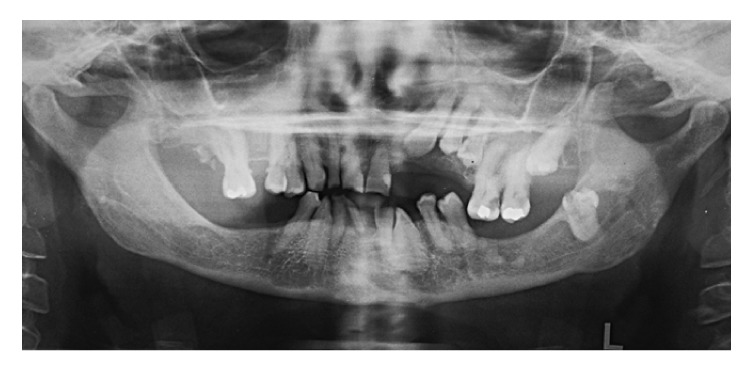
Orthopantomography revealed multiple dental anomalies: hypodontia, radicular fusion of 3.2–3.3, and impacted permanent teeth.
